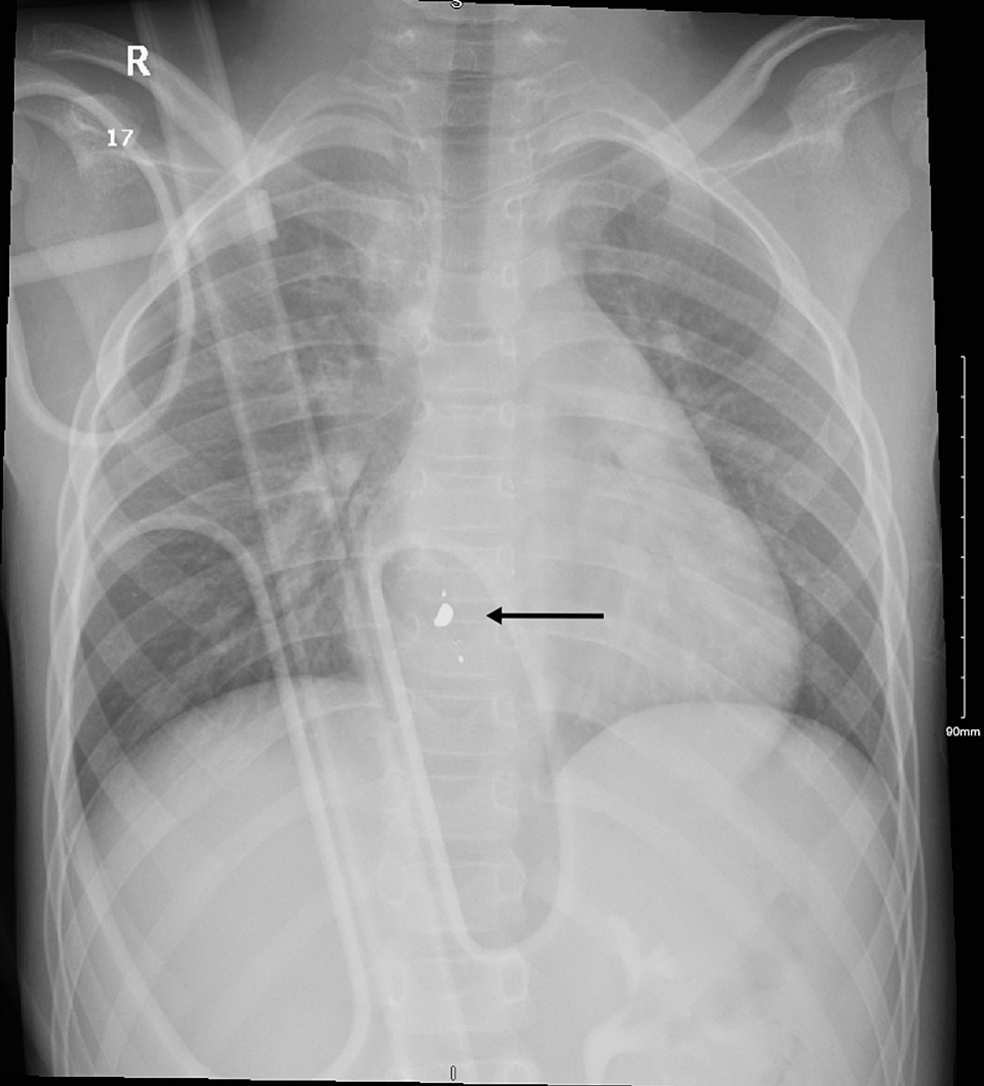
Chest X-ray showing foreign body representing the fragmented bullet.
